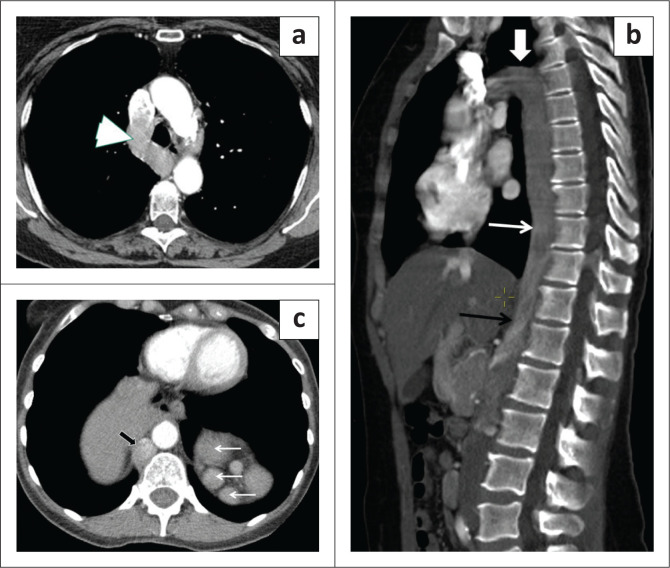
A 61-year-old female with a history of a colorectal mass on colonoscopy underwent CT chest, abdomen and pelvis for staging. (a) Post-contrast axial CT image shows a dilated azygos vein. (b) Sagittal oblique CT image shows the inferior vena cava (IVC) continuing into the thorax as the dilated azygos vein (white thin arrow) and arching (white thick arrow) in the superior mediastinum to drain into the superior vena cava. The black arrow represents the site of mixing of denser contrast from the renal vein. (c) Axial CT image at the level of aortic hiatus demonstrates azygos continuation of the IVC (black arrow) with polysplenia (white arrows) and mesocardia. The patient also had a midline liver and intestinal malrotation (not shown) suggestive of a left isomerism type of heterotaxy syndrome.
